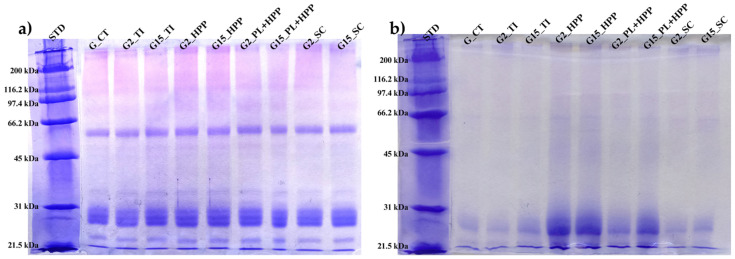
SDS-PAGE electrophoresis of Garnacha Blanca wine proteins after 2 (G2) and 15 days (G15) of contact with YDs (a) before heat test; (b) after heat test. CT: control untreated wine; SC: *S. cerevisiae* derivative; TI: Thermally Inactivated *S. ludwigii* derivative; HPP: High-Pressure Processed *S. ludwigii* derivative; PL + HPP: Partially Lysed + High-Pressure Processed *S. ludwigii* derivative.
